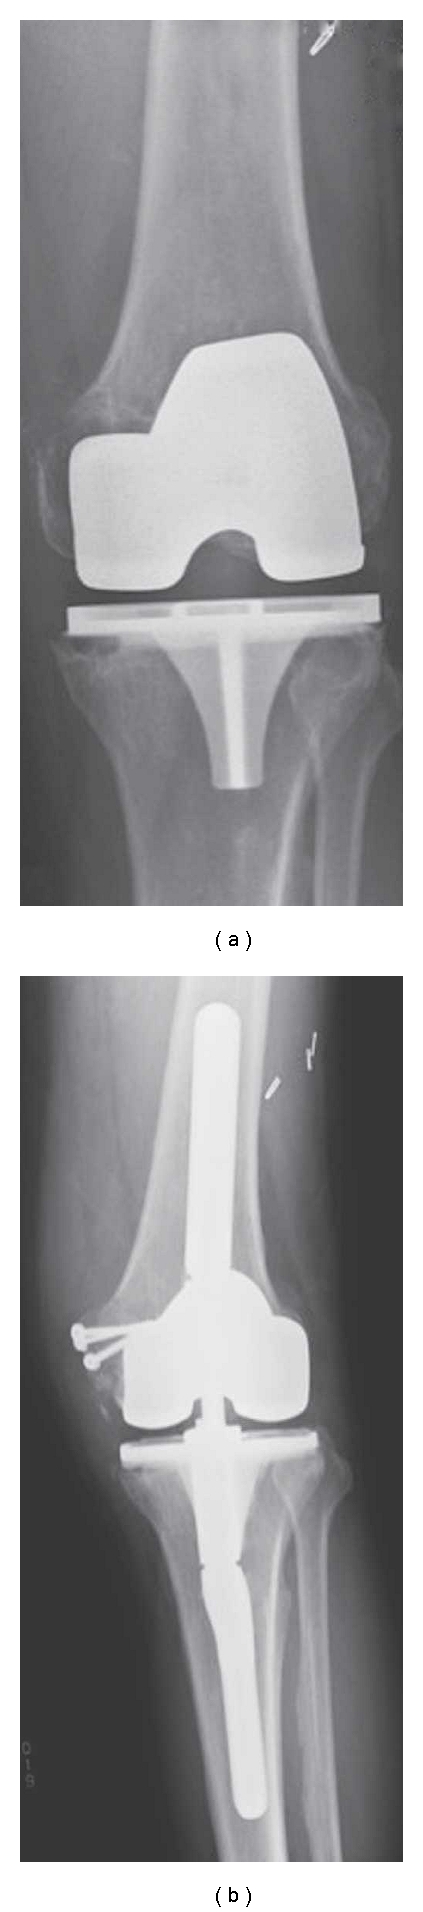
A radiograph shows uncontained bone loss in the medial femoral condyle secondary to osteolysis (a). A radiograph showing revision TKA with reconstruction of the medial femoral condyle using structural allograft fixed with screws (b).
